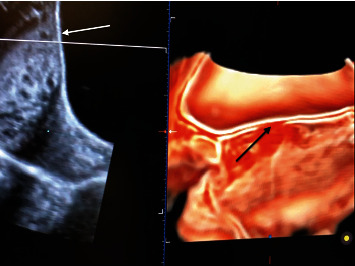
3D ultrasound image. White arrow indicates normal bladder line; black arrow indicates the perimetrial interruption.
